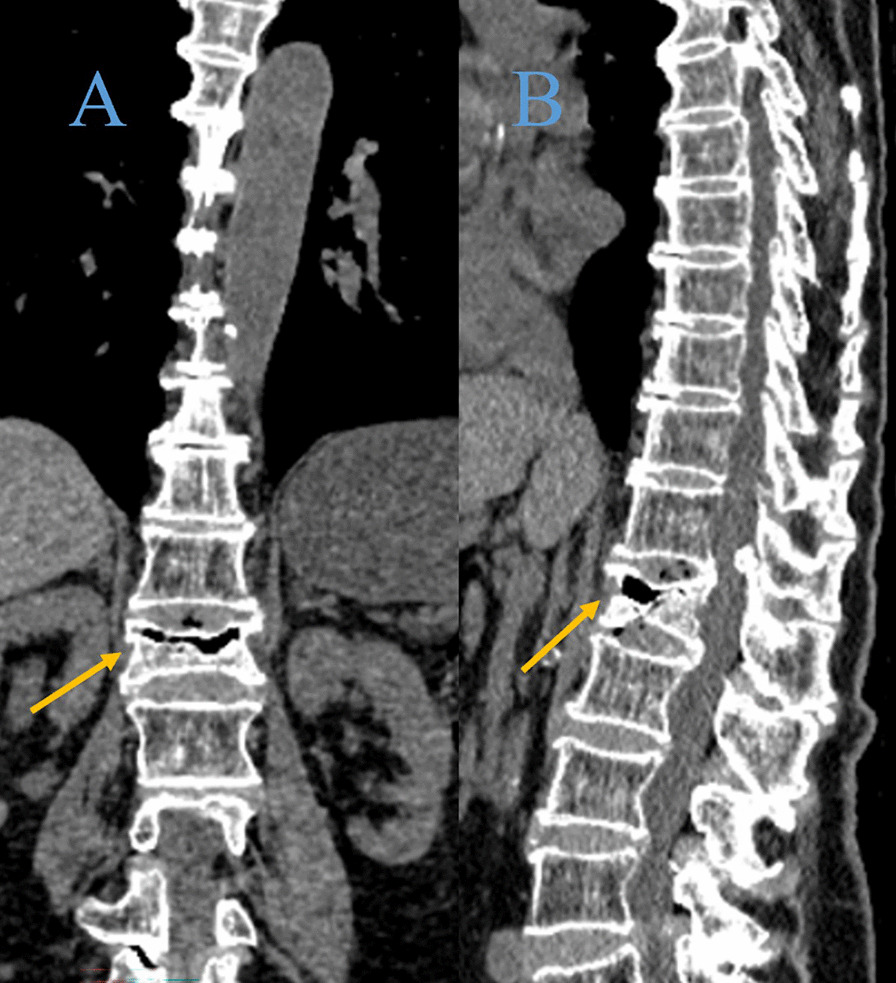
Intravertebral vacuum cleft was visible on coronal (A, yellow arrow) and sagittal (B, yellow arrow) views of CT
